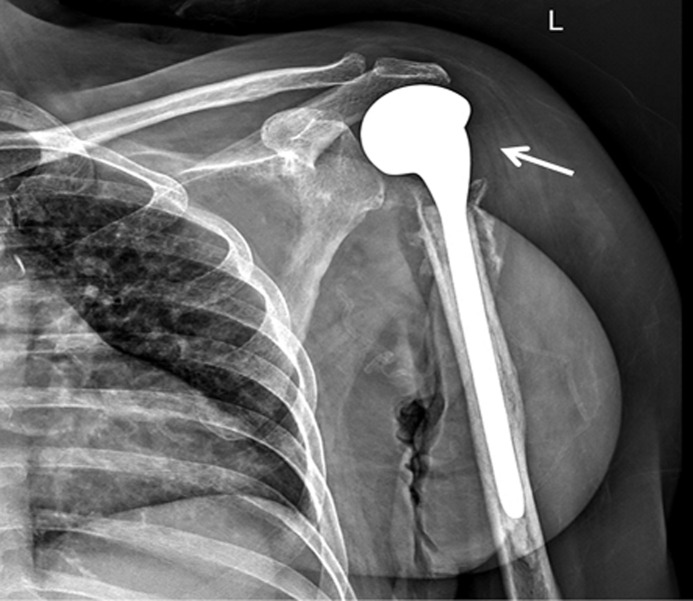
postoperative X-ray of left shoulder at 6 months follow-up showing hemi replacement implant in situ; there are no new lesions or any signs of implant loosening
